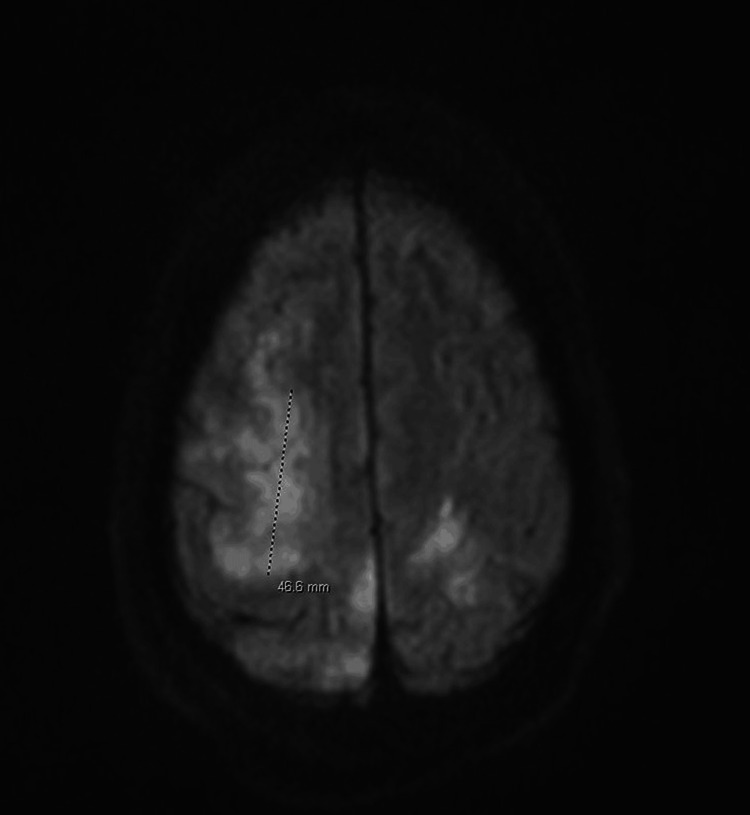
MRI findings suggestive of acute embolic stroke
